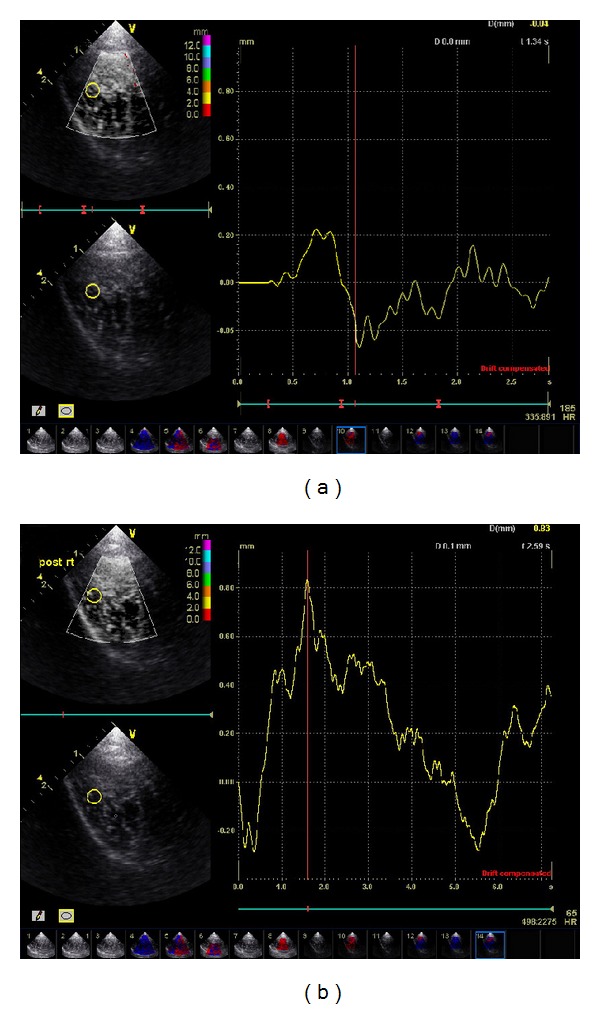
Tissue displacement on acupoints following needle stimulation before and after* de qi*. In vivo ultrasonic imaging using a System FiVe (Vingmed) at 7.5 MHz was performed on the healthy subjects at different stages of acupuncture needle stimulation including before* de qi* and during* de qi*. Displacements were estimated using the ultrasonic radio-frequency (RF) data. Seventy RF scans were acquired continuously during each experiment at the rate of 13.2 frames per second.
